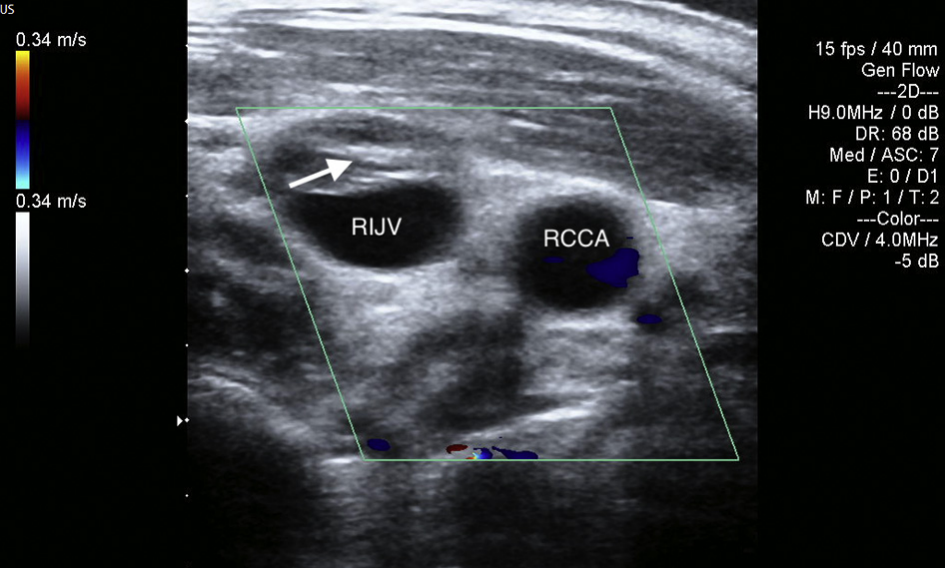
Partial resolution of thrombi in the right internal jugular vein (*arrow*) on day 7 following anticoagulant therapy on postoperative carotid ultrasound.
